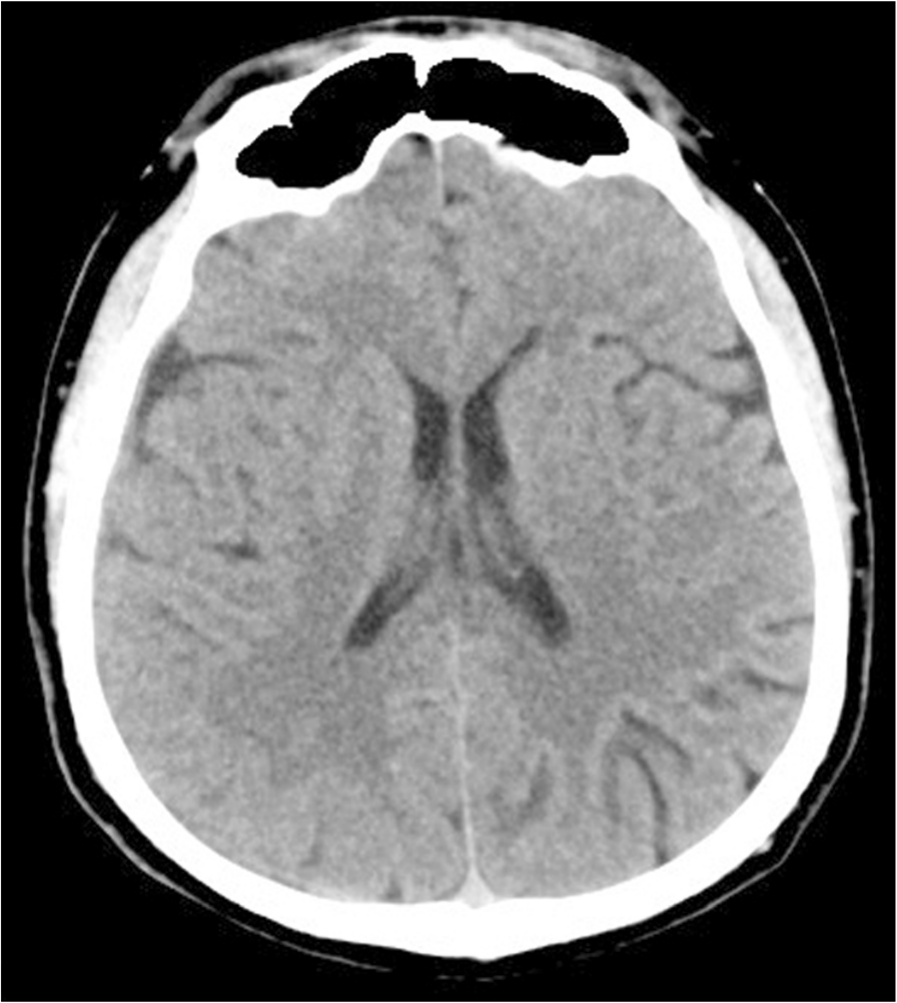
Head CT shows spontaneous resolution of the CSDH. CT: computed tomography; CSDH: chronic subdural hematoma
